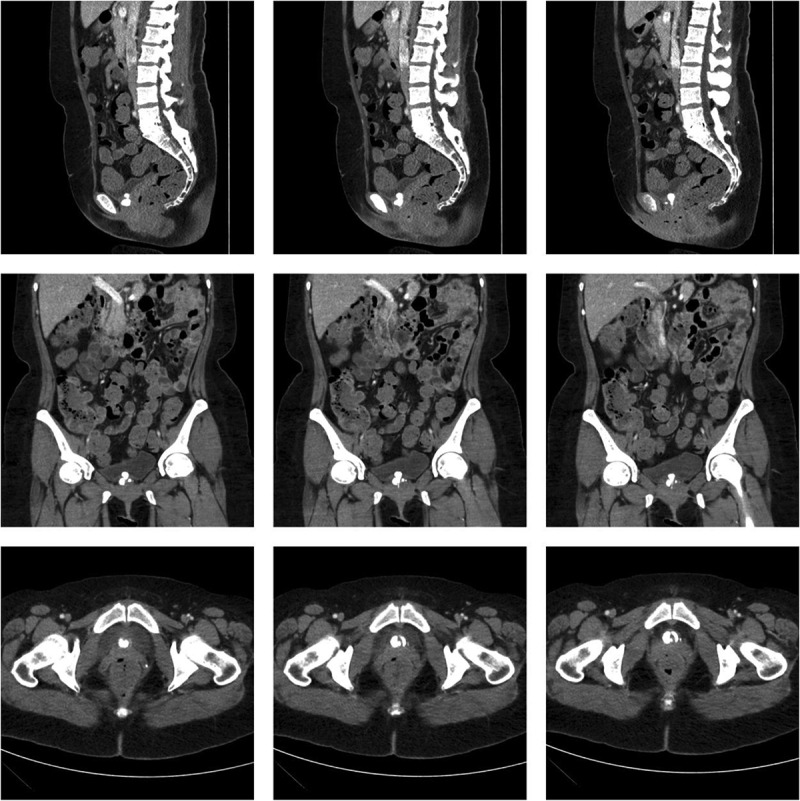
Sagittal, coronal, and axial CT scans of the abdomen and pelvis showing the foreign body granuloma, which can be misinterpreted as a bladder calcification or stone.
